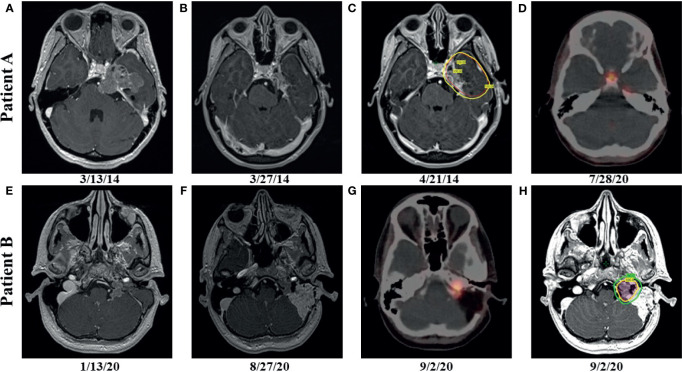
Patient A: preoperative, axial T1 postcontrast weighted magnetic resonance (MRI) imaging showing enhancing disease (A); postoperative, axial T1 postcontrast weighted MRI imaging showing enhancing, residual disease (B); radiation therapy (RT) planning using volumetric arc therapy (VMAT) resulted in excellent coverage of the planning target volume (PTV) (red) by the 100% isodose line (yellow) corresponding to 5940 cGy (C); follow up gallium-68 dotatate positron emission tomography (PET) after more than 6 years showing hypermetabolic, recurrent disease in the left tentorial leaflet and physiologic uptake in the pituitary (D); Patient B: preoperative, axial T1 postcontrast weighted MRI imaging showing enhancing disease (E); postoperative, axial T1 postcontrast weighted MRI imaging showing enhancing, residual disease (F); postoperative PET showing hypermetabolic, residual disease (G); RT planning using VMAT resulted in excellent coverage of the 6000 cGy (red) and 5400 cGy (blue) PTVs by the 100% (yellow) and 90% (green) isodose lines, respectively (H).
